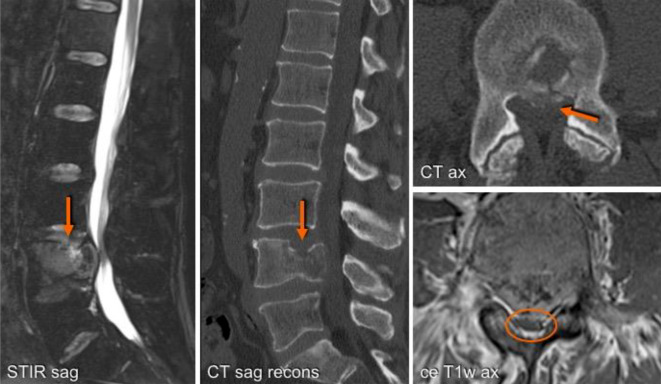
An L4 vertebral lesion replacing fatty marrow mass with an intraspinal portion but no pedicle infiltration. On the sagittal T2-weighted WI-STIR (short-TI inversion recovery weighted imaging) sequence in this 63-year-old patient with metastatic urothelial carcinoma both the hyperintense signal infiltration and, when compared with the CT scan, the pathological fracture are more conspicuous. Axial reconstruction (*CT*) reveals an intraspinal bone fragment with compression of the dural sac, which is best delineated on the contrast-enhanced axial T1-weighted sequence (*ce T1w ax*)
